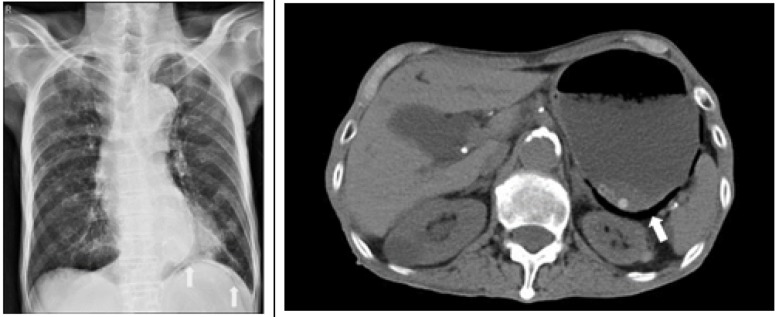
Posterior-anterior chest X-ray (left) and coronal view of abdominal computed tomography scan (right) of patient
